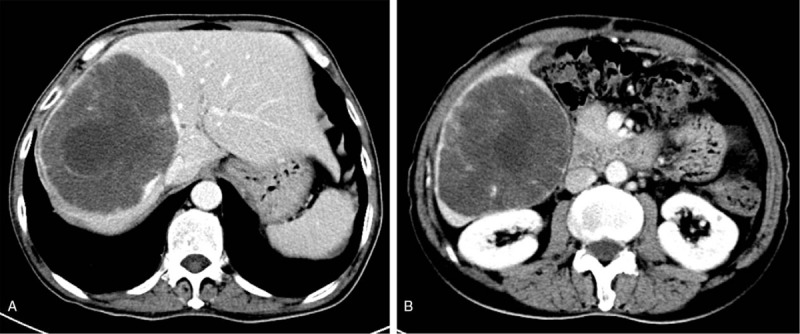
Tumor size (longest diameter × shortest diameter) on computed tomography (1 month after operation). (A) 10.3 × 13.2 cm. (B) 11.3 × 9.6 cm.
